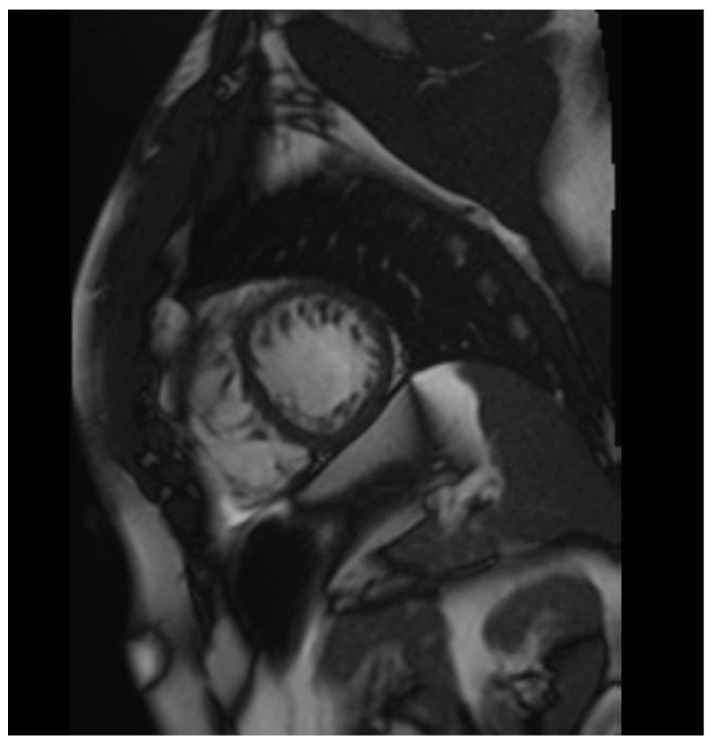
E.M. Cardiac MRI. SSFP cine sequence. Short-axis view, mid-segments. The image shows left ventricular non-compaction. MRI: magnetic resonance imaging. SSFP: steady-state free precession.
